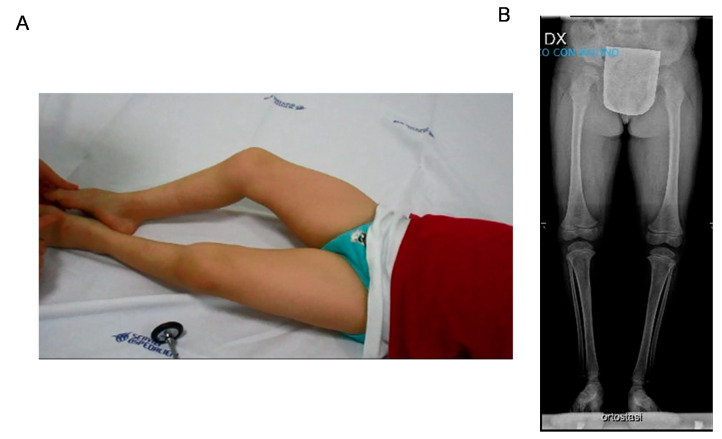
(A) Patient at rest aged 9 years. Lower limb stiffness with bent knees and hip posture is evident. Increased muscle bulk of tight muscles is also pictured. (B) X-rays of lower limbs in standing position showed shallow hip acetabulum, flattened femoral epiphyses, and slender diaphysis of the tibia.
